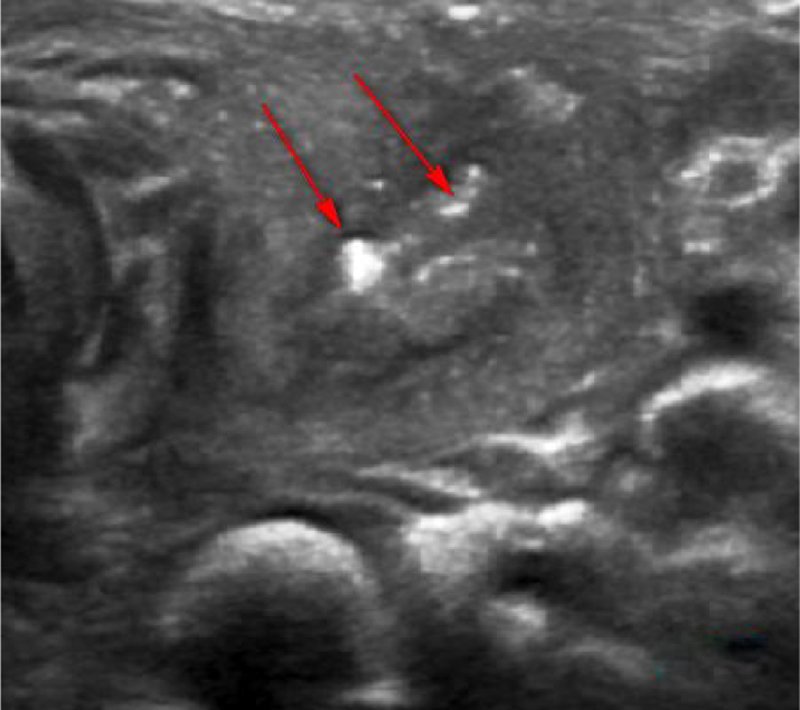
Ultrasound examination: strong echoes of gas scattered in the left thyroid abscess.
